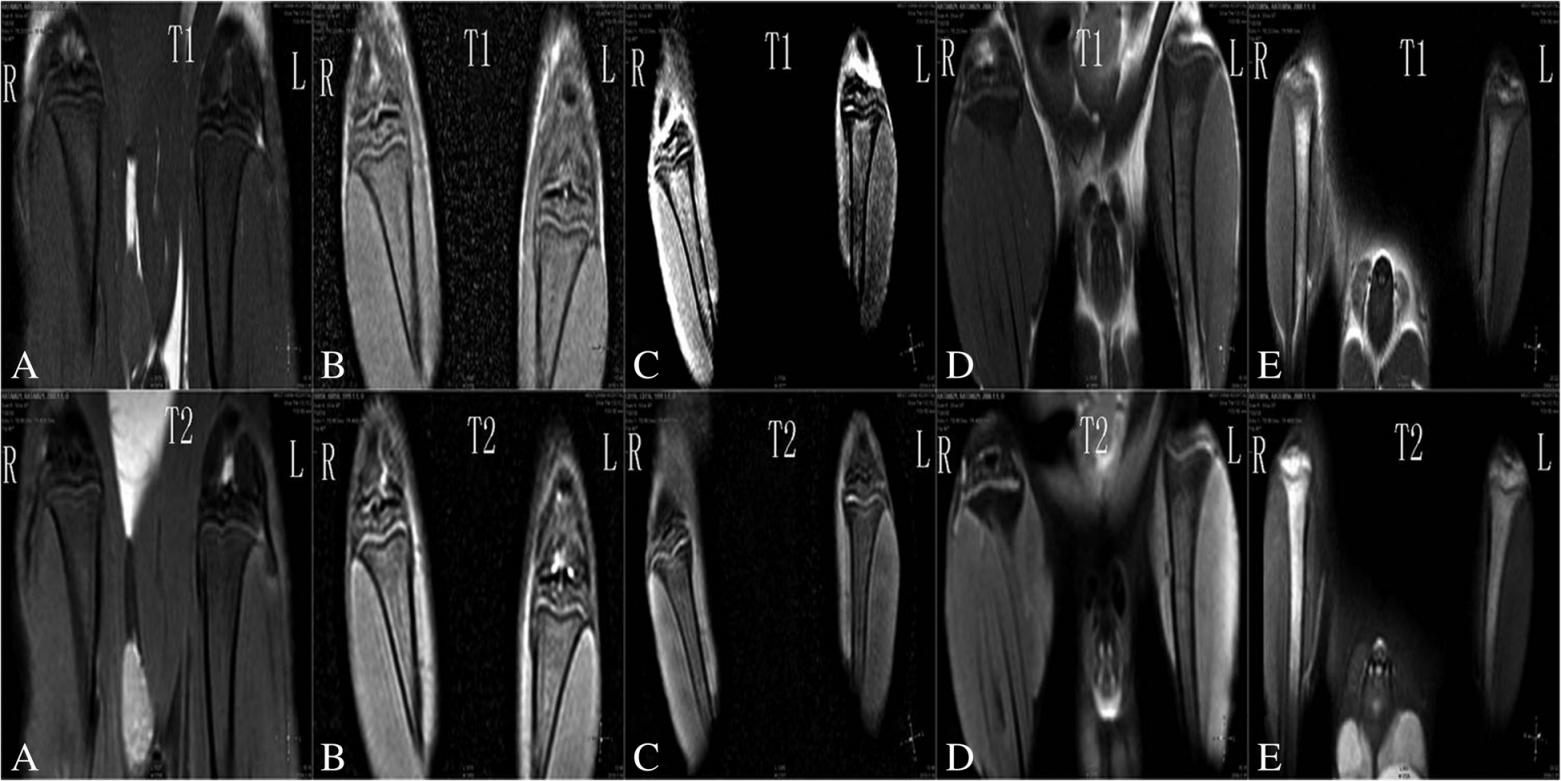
MR images of the epiphyseal plate at 8 weeks. (A) Epiphyseal plate *shows clear, wide, continuous and smooth; the intensity shows medium and uniformity*. (B) Epiphyseal plate* shows clear, narrow, continuous and smooth; the intensity shows medium and uniformity*. (C) Epiphyseal plate *shows narrow, disccontinuous and rough; the intensity shows higher or low and uneven*. (D) Epiphyseal plate *shows clear, wide, continuous and smooth; the intensity shows medium and uniformity*. (E) Epiphyseal plate *shows narrow, discontinuous and rough; the intensity shows higher or low and uneven*.
